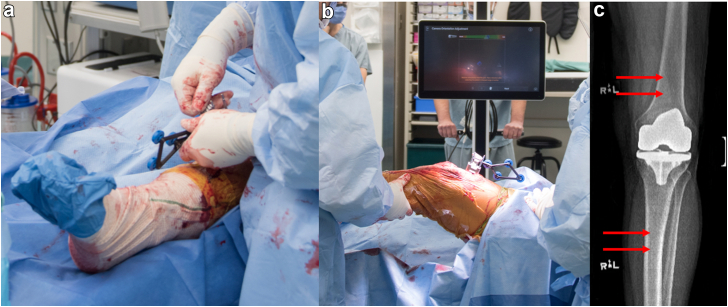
(a) Intraoperative photograph demonstrating the positioning of the tibial array and pins during imageless robotic-assisted rTKA. (b) Intraoperative view showing the placement of the femoral array and the navigation system interface verifying real-time capture and visibility of both arrays. (c) Preoperative radiograph of a left TKA before the rTKA surgery. The pin incisions, marked with red arrows, indicate the pin locations used to guide accurate alignment and component placement during the procedure.
